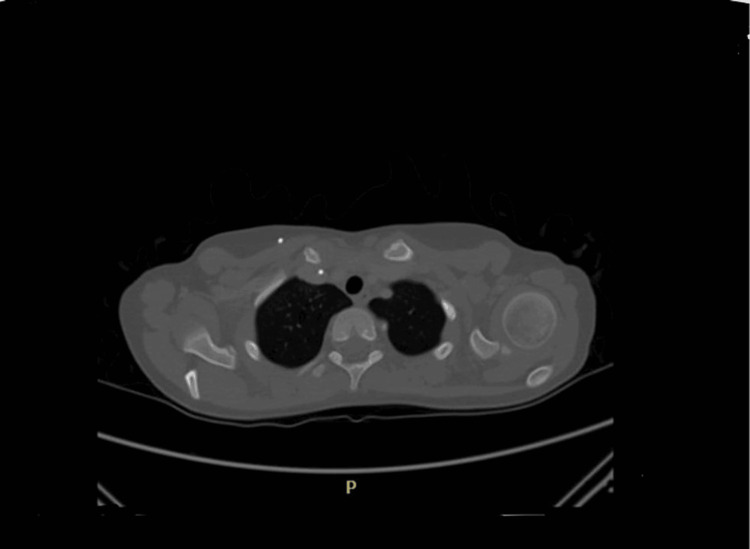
Computed tomography scan of the left scapula post-chemotherapy showing significant interval regression in the size and appearance of the left scapular soft-tissue mass lesion
